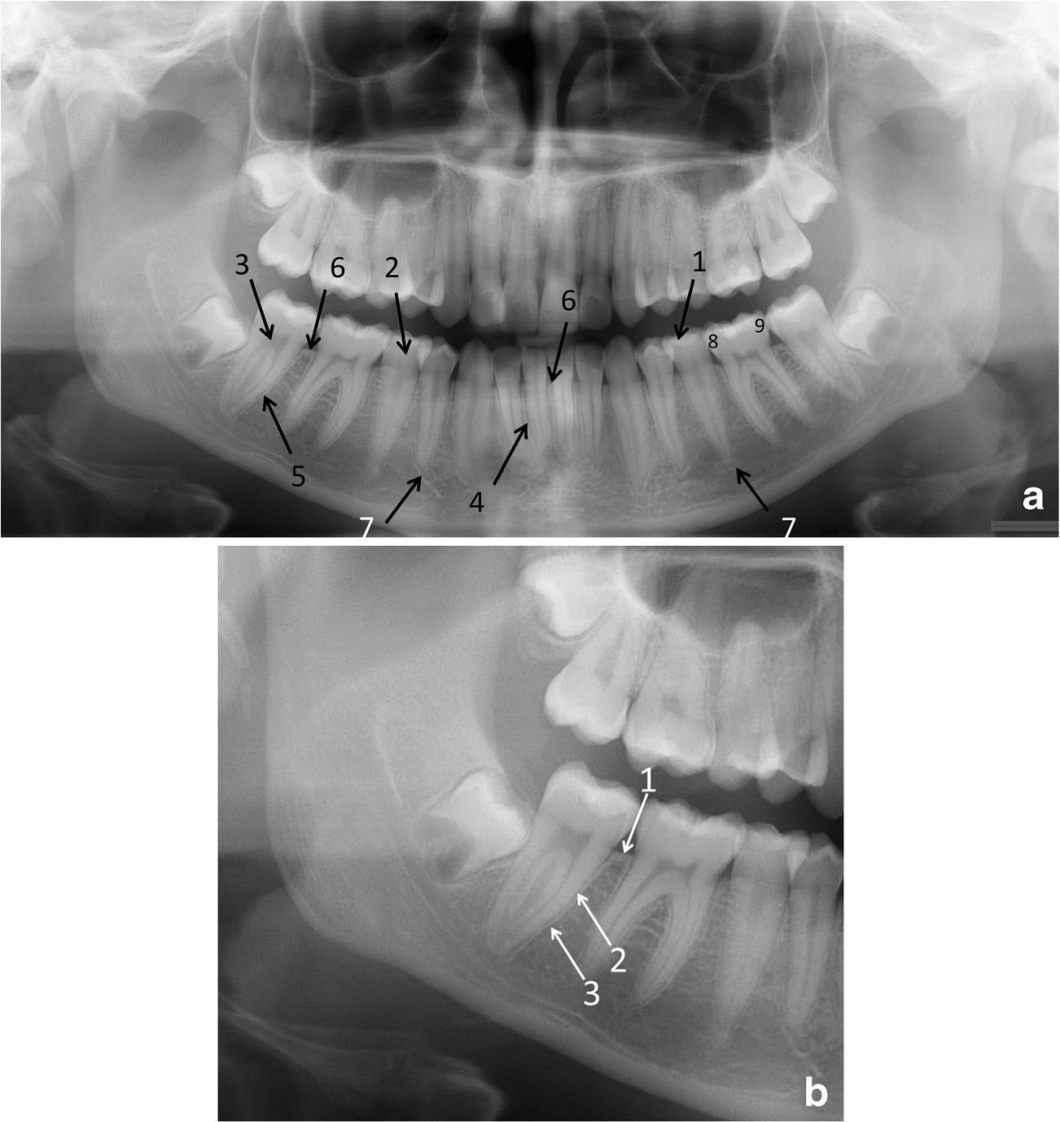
a Fine anatomical structures of teeth and their supporting structures in a PTG and b in a part of a PTG image: (a) 1, enamel; 2, dentine; 3, pulp chamber; 4, periodontal ligament space (fine radiolucent line around the root); 5, lamina dura (fine radiopaque line around the root); 6, crestal margin; 7, periapical area; 8, mesial side of the tooth; 9, distal side of the tooth. (b) 1, crestal margin; 2, periodontal ligament space; 3, lamina duraEach tooth should be evaluated for the presence of caries, restorations, number and morphology of the root, root filling and resorptions. The apical and periodontal tissue will be checked for the integrity, continuity, width and thickness of the radiolucent line around the root (periodontal ligament space) and the radiopaque line (lamina dura) (Fig. 6), any associated radiolucent or radiopaque finding next to the apex of the tooth, the pattern and density of the surrounding bone, the level and quality of the crestal bone, integrity of the corticated crestal margin, signs of horizontal or vertical bone loss, any calculus deposits and signs of bone loss in the furcation area (Fig. 7). However, supplementary intraoral radiographs are often indicated for example for the apical and the periodontal tissue evaluation and for caries diagnostics.
